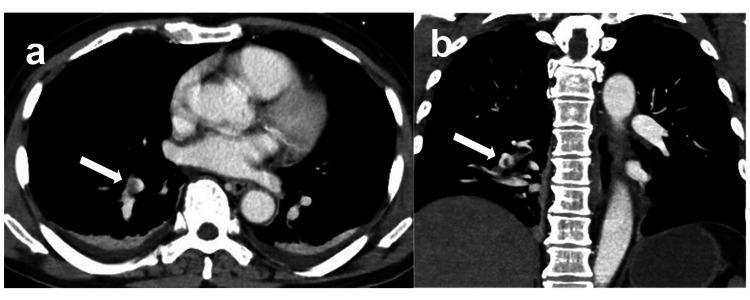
Postoperative contrast-enhanced CT findings of the torso. a: Contrast-enhanced axial CT shows a thrombus in a branch of the right pulmonary artery (arrow). b: Contrast-enhanced coronal CT shows a thrombus in a branch of the right pulmonary artery (arrow).
